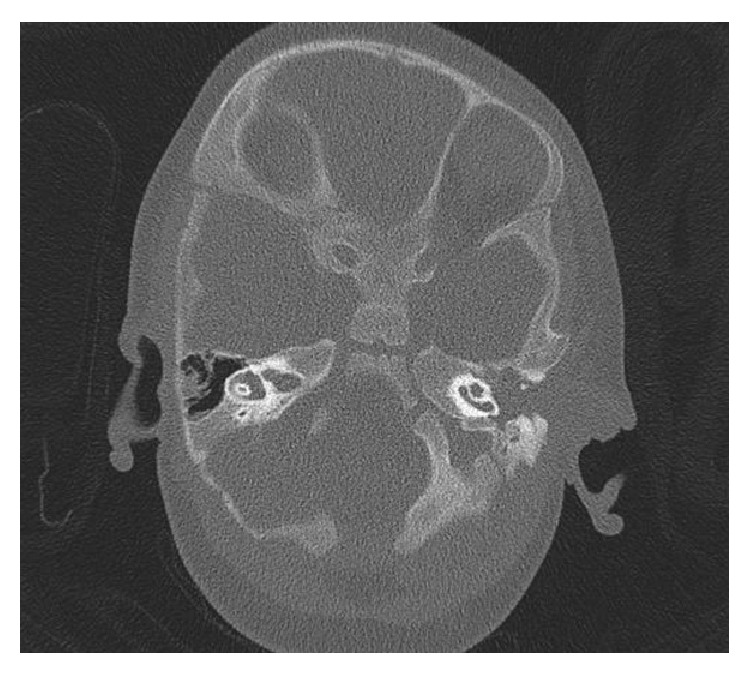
Head CT scan (axial) shows large homogeneous in the left temporal bone.
